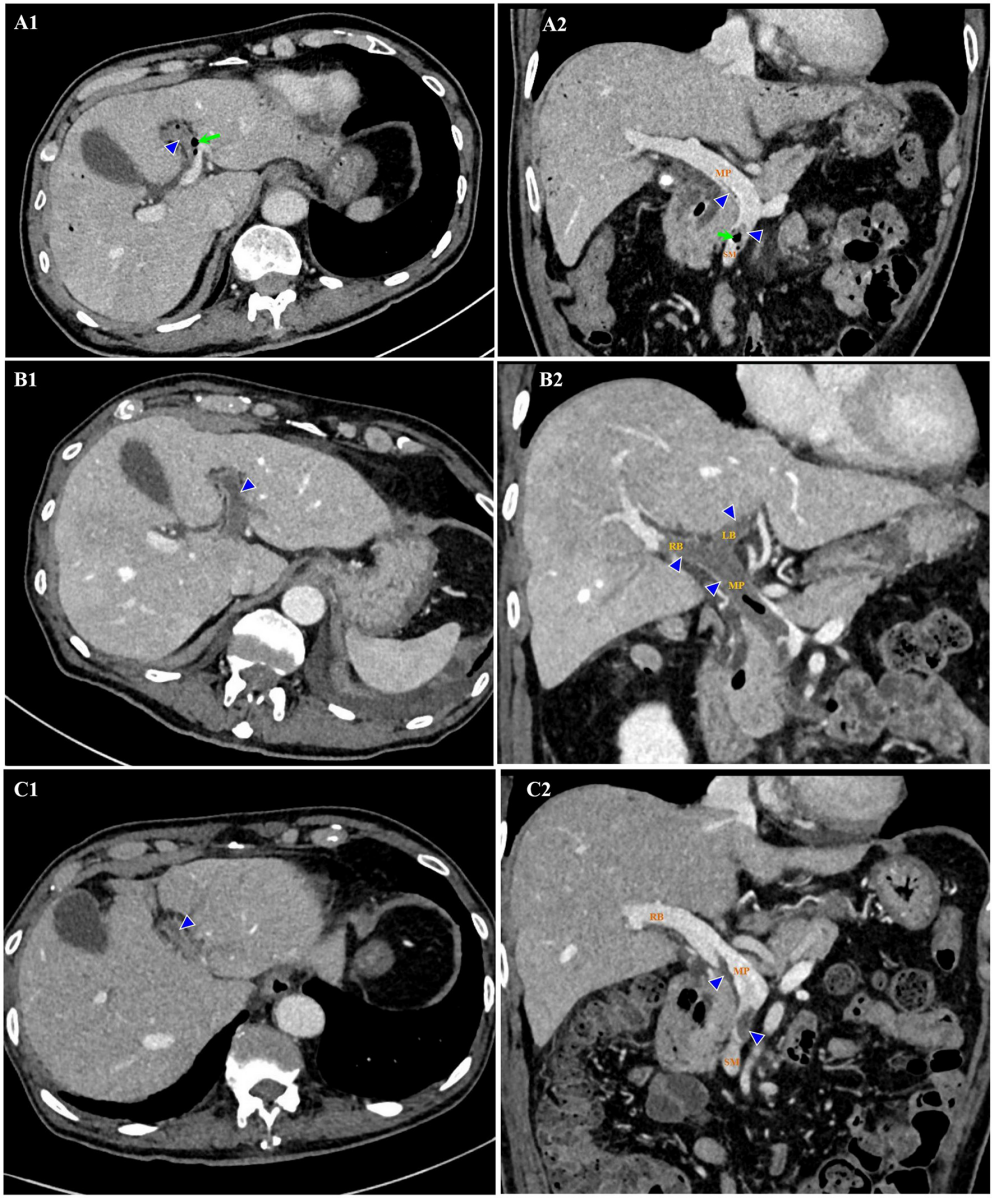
Thrombosis (triangles) and gas (arrows) in the superior mesenteric and portal veins on contrast-enhanced CT. Hospital day 2 (February 14, 2020). Thrombosis and gas in the LB of portal vein on axial view (A1). Thrombosis in the SM and MP, and gas in the SM on coronal view (A2). Hospital day 12 (February 24, 2020). Thrombosis enlarged in the extended LB of portal vein on axial view (B1). Thrombosis enlarged in the MP, LB, and RB of portal vein, with gas in the MP on coronal view (B2). Hospital day 23 (March 6, 2020). Thrombosis shrank in the LB of portal vein on axial view (C1). Thrombosis shrank in the SM, MP, and RB of portal vein on coronal view (C2). No gas was detected. CT indicates computed tomography; LB, left branch (of portal vein); MP, main portal vein; RB, right branch (of portal vein); SM, superior mesenteric vein.
